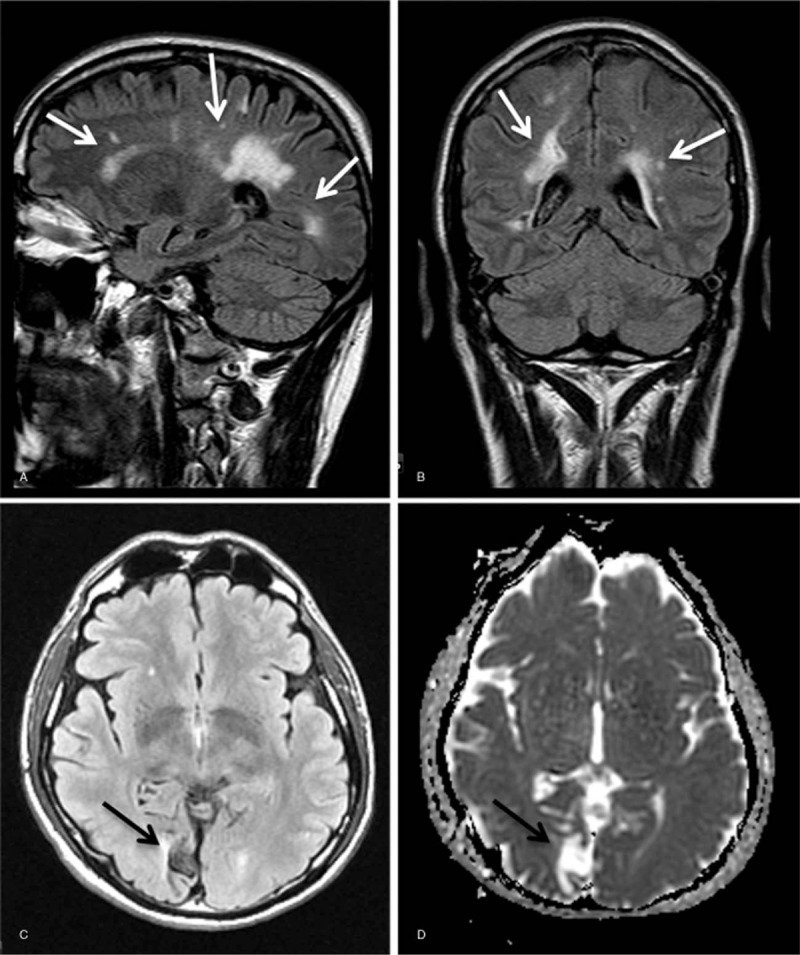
Representative examples of performed brain MRI investigations for evaluation of brain tissue involvement in patients with hemizygous -10 C>T single nucleotide polymorphisms. Brain MRI reveals micro- and/or macrovasculopathy (A and B, patient no. 12, white arrows) and/or ischemic encephalopathy (C and D, patient no. 13, black arrows). MRI = magnetic resonance imaging.
